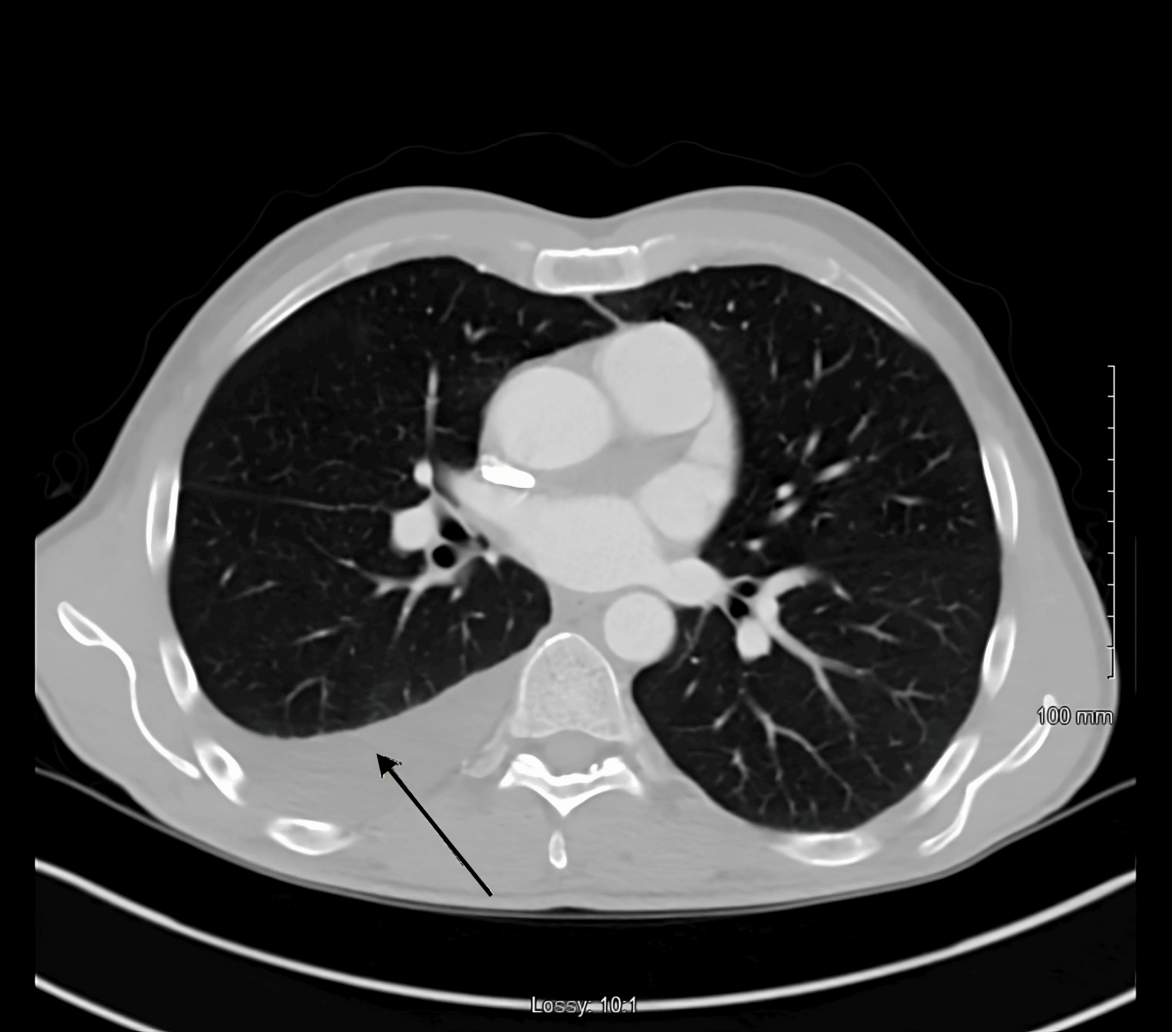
CT chest showed loculated pleural effusion on the left side and trace pleural effusion on the right (black arrow)
